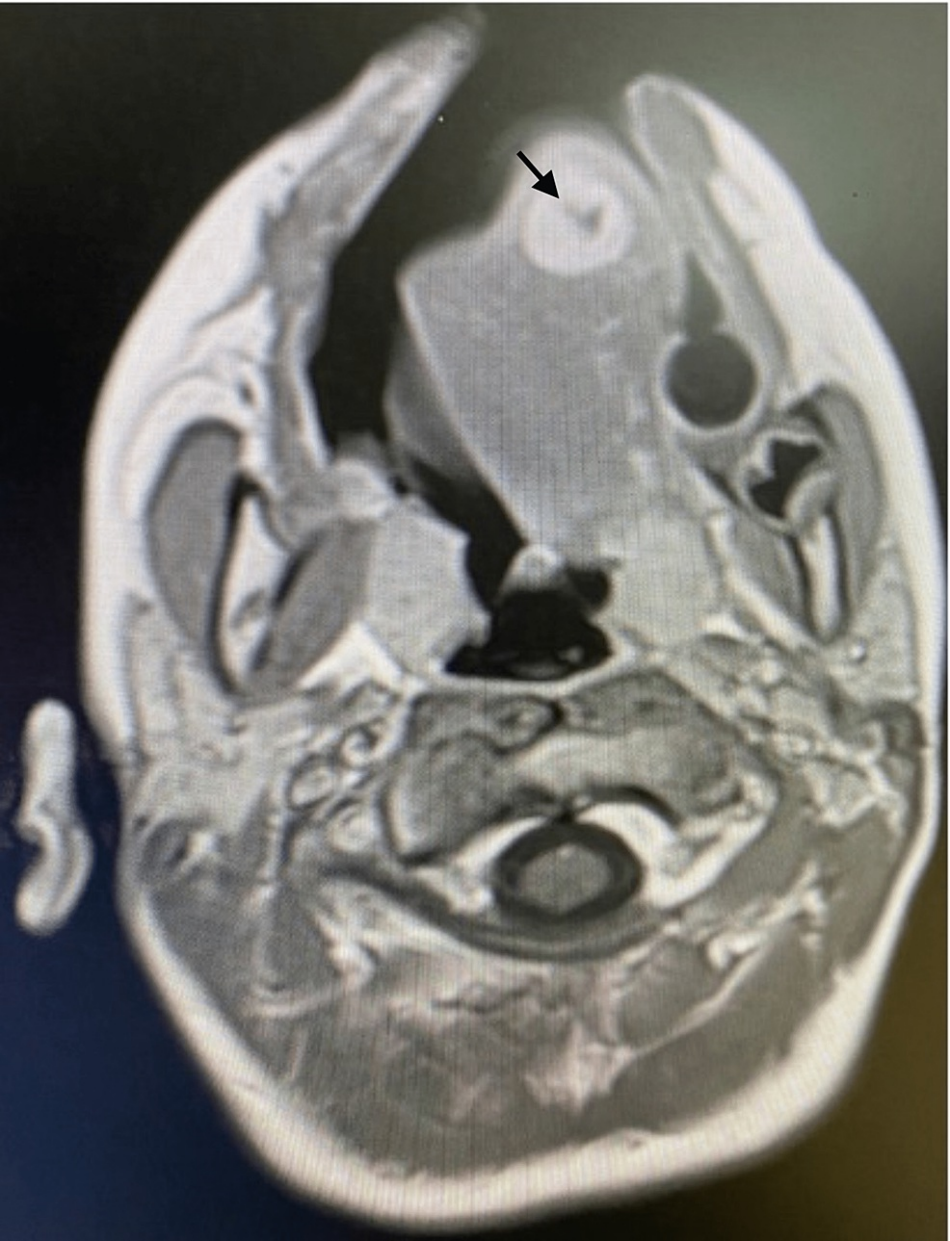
Axial T1 post-contrast magnetic resonance imaging (MRI) of the head demonstrates a peripherally enhancing mass measuring 1.3 x 1.4 cm (black arrow) with central cystic/necrotic component.
